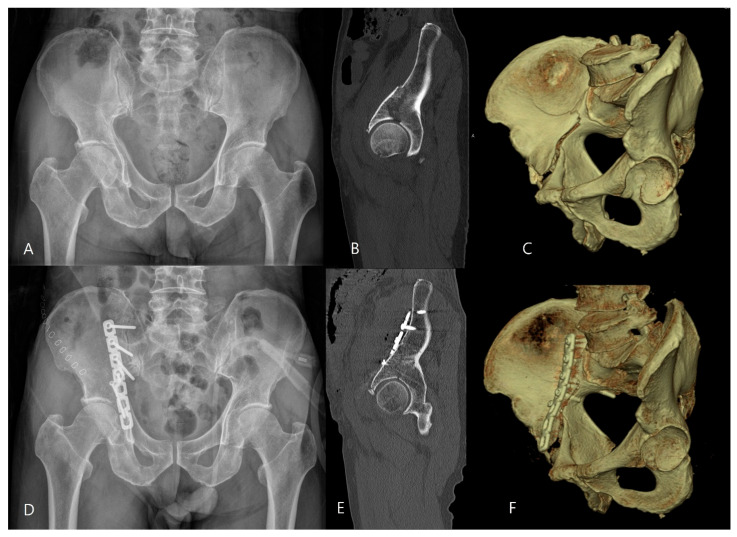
Preoperative images. (A) Anteroposterior radiograph of a right acetabular fracture, (B) a sagittal reconstruction of computed tomography (CT) scan (B), and three-dimensional CT scan (C) showing an incomplete anterior column fracture not involving the quadrilateral plate. Postoperative anteroposterior radiograph (D) of the anterior column fracture stabilized with a 4.5 mm reconstruction plate. Postoperative sagittal reconstruction (E) and three-dimensional CT scan (F).
